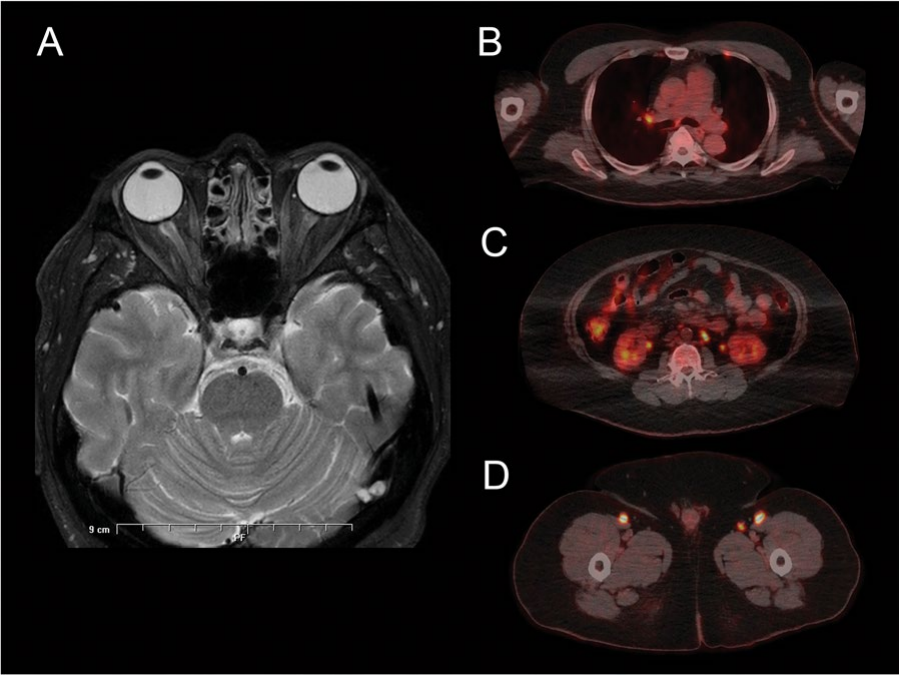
A Magnetic resonance imaging of the orbits (axial section, T2-weighted) reveals increased intraneural signal of the right optic nerve. B Positron emission tomography (axial section) detects an increased focal 18-Fluodeoxygluocse uptake in the right hilar lymph node. C Intense uptake in bilateral para-aortic and left infra-renal lymph nodes. D Intense uptake in bilateral inguinal and femoral lymph node regions
